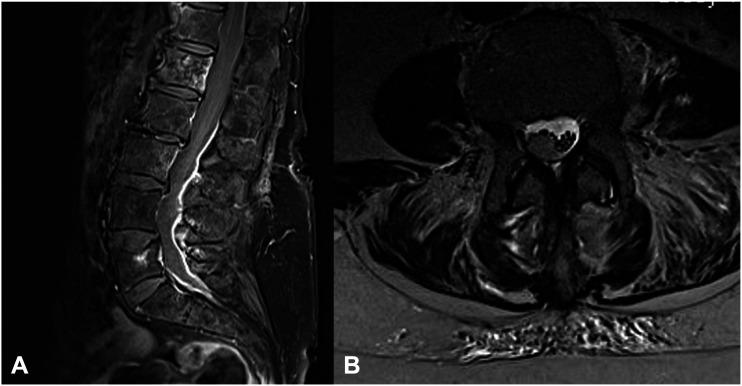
Postcontrast sagittal (A) (T1 gadolinium sequence) and T2-weighted axial (B) magnetic resonance imaging demonstrating pronounced meningeal enhancement and clear intramedullary compression with ring enhancement collection extending from L2-S1 in a65-year-old male patient presenting with lumbar pain and progressive lower extremity weakness.
